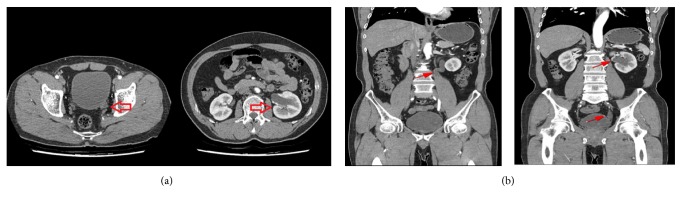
Postoperative follow-up imaging of cystographic computed tomography showing no leakage at anastomosis of ureteroneocystostomy with ureteral stent in situ.
